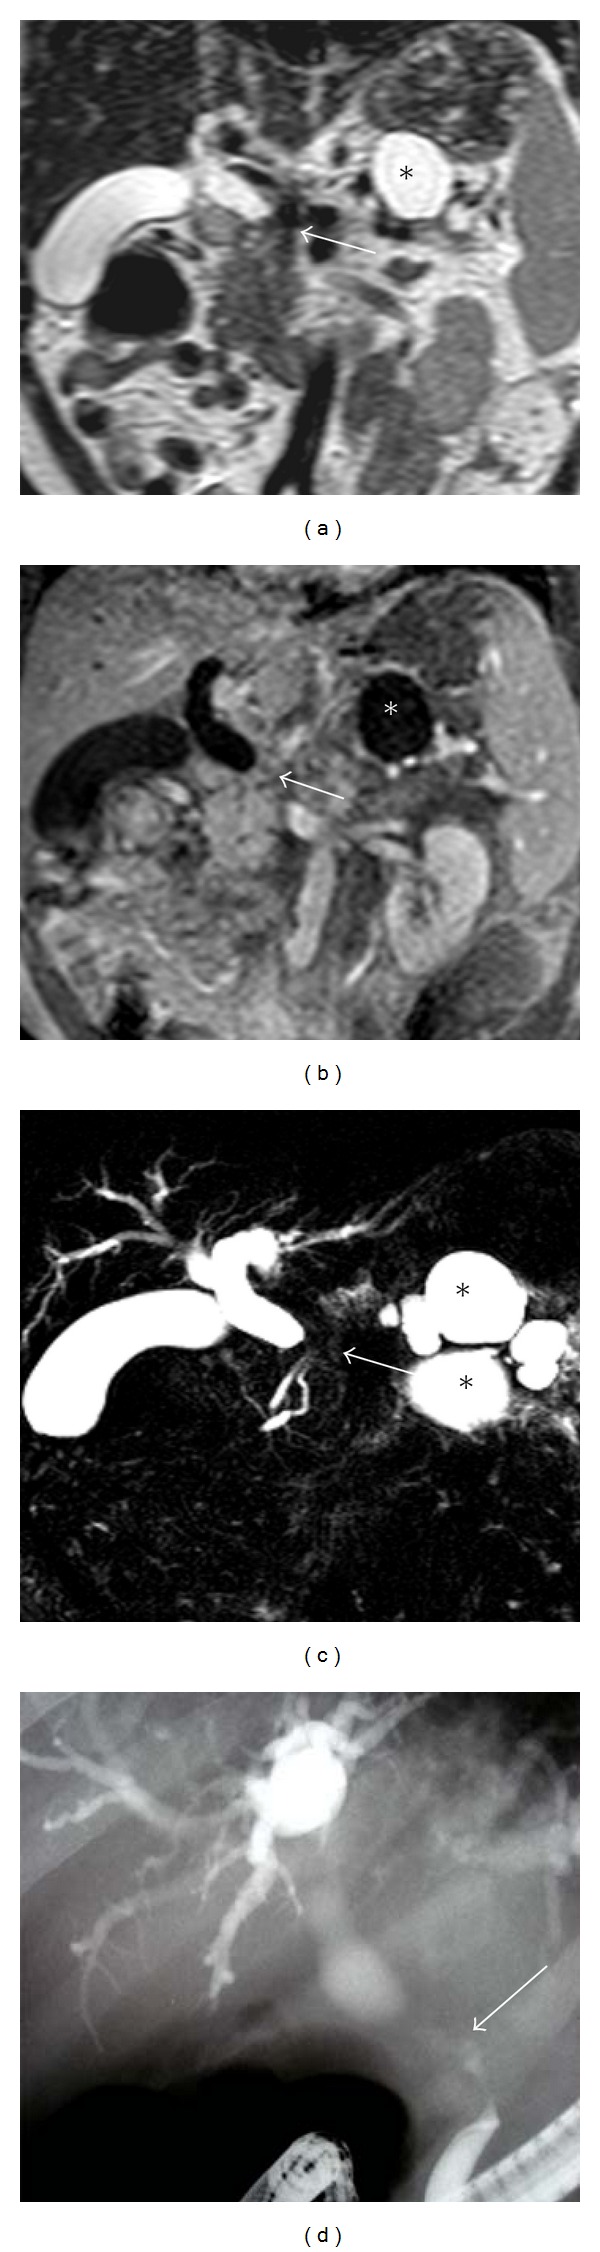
T2-weighted (a), contrast-enhanced T1-weighted MRI (b), and MRCP slices demonstrate significant stenosis in the common bile duct at the level of pancreas head (white arrow) and bile duct dilatation proximal to the stenosis. Any mass lesion is not seen at the stenosis level. On MRI and MRCP, the cause of stenosis considered as chronic pancreatitis because of pseudocysts located in corpus and tail of the pancreas (asterisk). (d) ERCP demonstrates the malignant character of stricture (black arrow), and the result of biopsy was adenocarcinoma of the pancreas.
